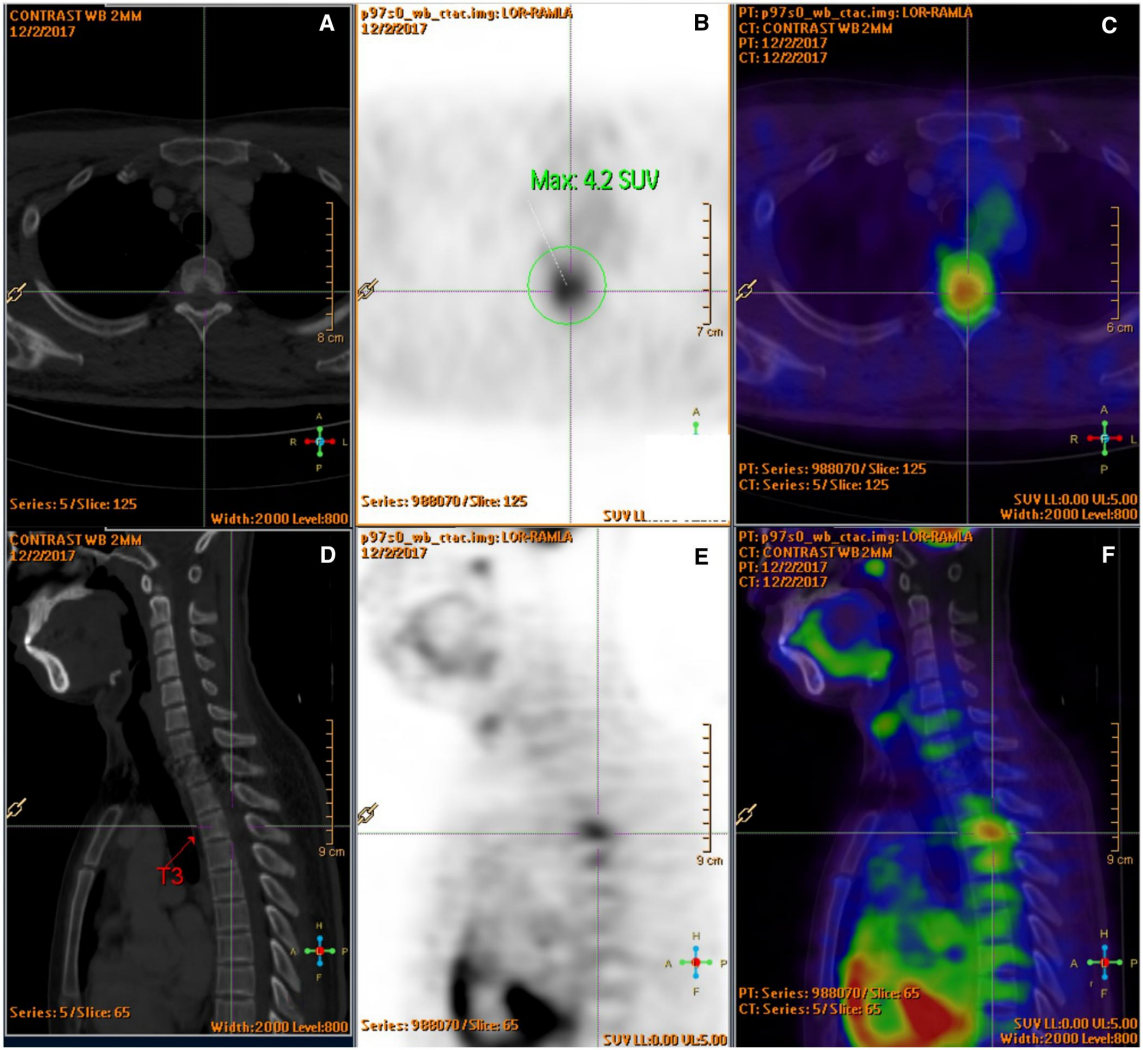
(A-F) F-18 FDG PET-CT showed an intraspinal mass at T3 level with increased FDG uptake (SUV max 4.2). No overt bone erosion or extraspinal hypermetabolic lesion was detected.
